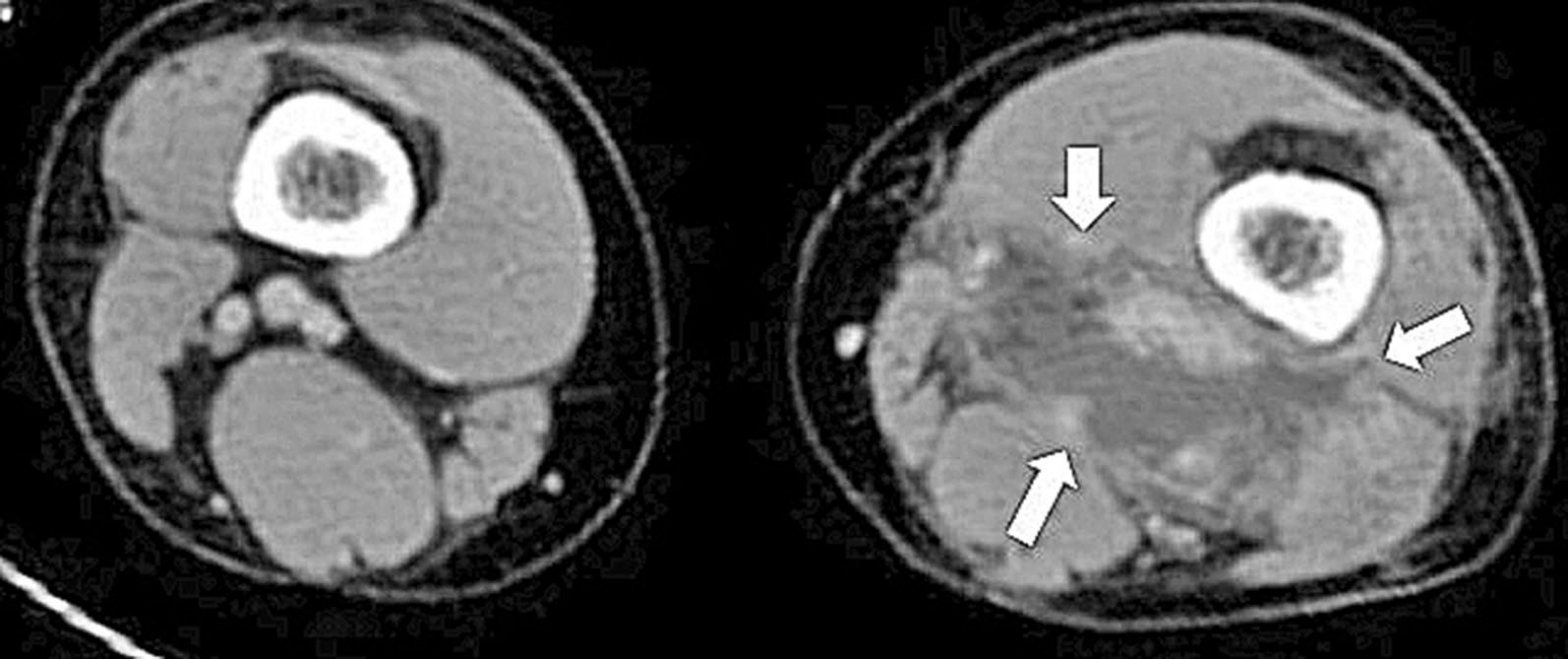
Enhanced computed tomography reveals fluid between the dorsal distal muscles of the *left thigh* and an enhanced margin of fluid (*white arrows*)
